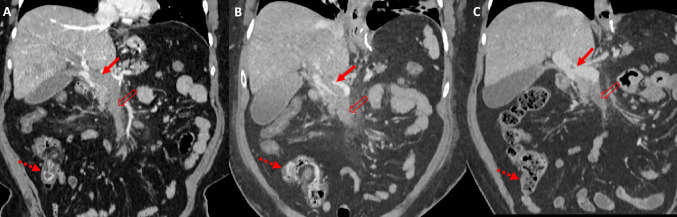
Case 2. Coronal reformats of CT examinations performed at day 0 (A), day 3 (B), and day 15 (C) post admission showing a progressive reduction in thrombus burden within the portal vein (solid arrow) post systemic thrombolytic and anticoagulant therapy. Note unchanged thrombus within the superior mesenteric vein (unfilled arrow), but improvement in the oedema of the caecum and ascending colon (dashed arrow)
